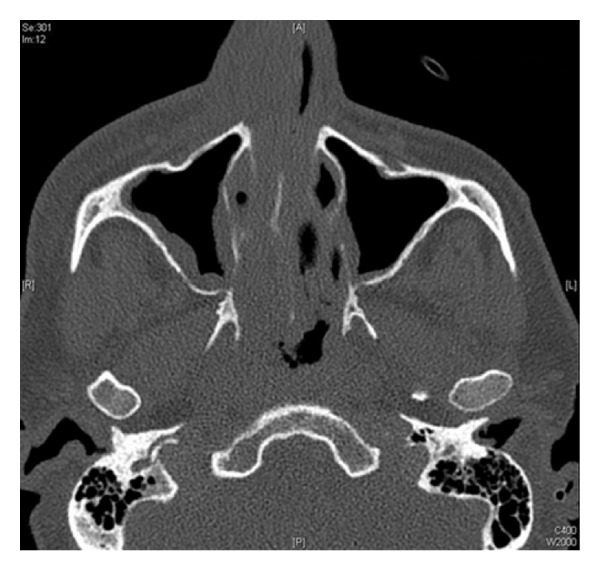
Sagittal CT view of sinuses showing thickening of the mucosa of the anterior right side septum and soft tissue thickening overlying the right side nose and cheek.
